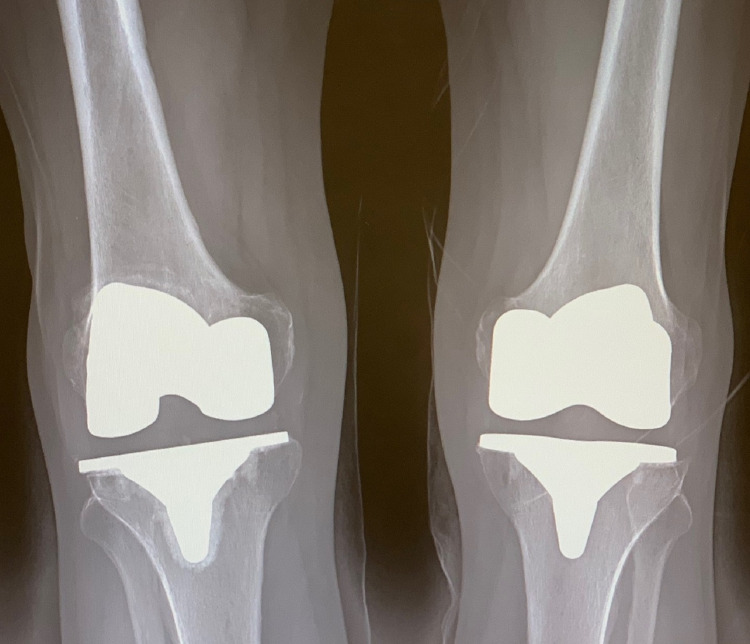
Standing AP preoperative radiograph of the bilateral knees demonstrating aseptic loosening with early mild cystic formation underneath the right tibial baseplate. AP: anteroposterior
